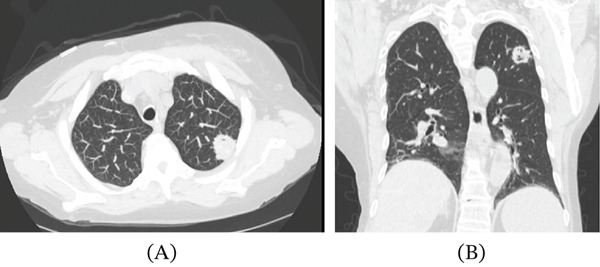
CT imaging of the chest demonstrating cavitary morphology of the lesion. (A) Axial CT image showing a cavitary lesion within the right upper lobe. (B) Coronal CT reconstruction demonstrating the cavitary nature of the lesion.
